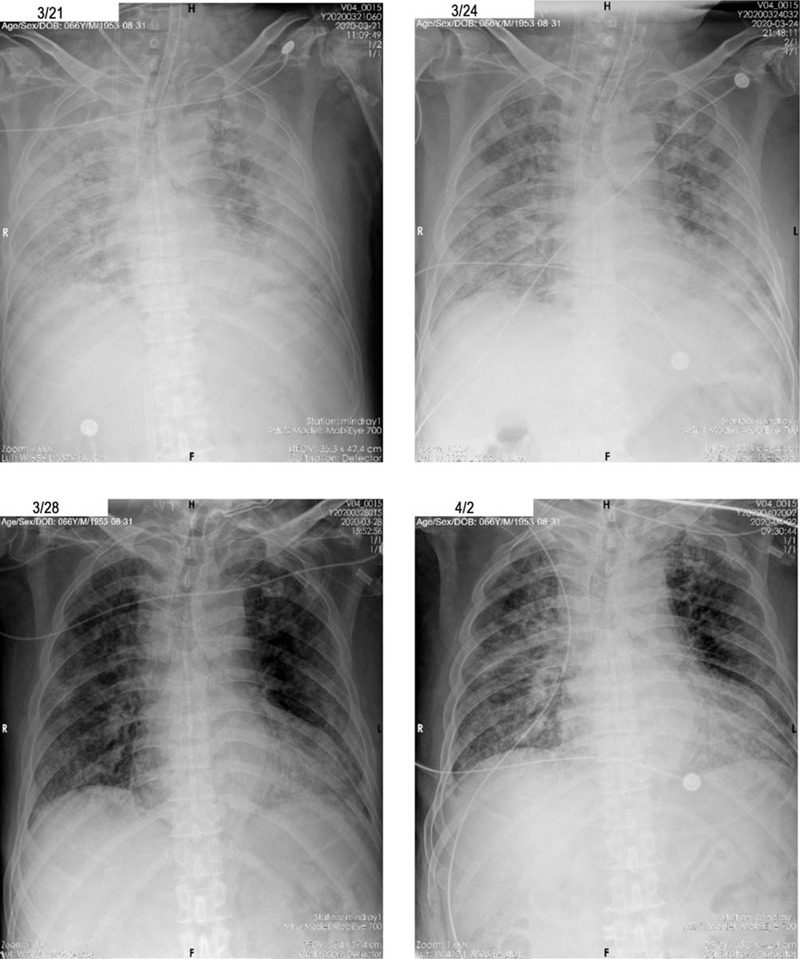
Chest X-ray images of the patient.
